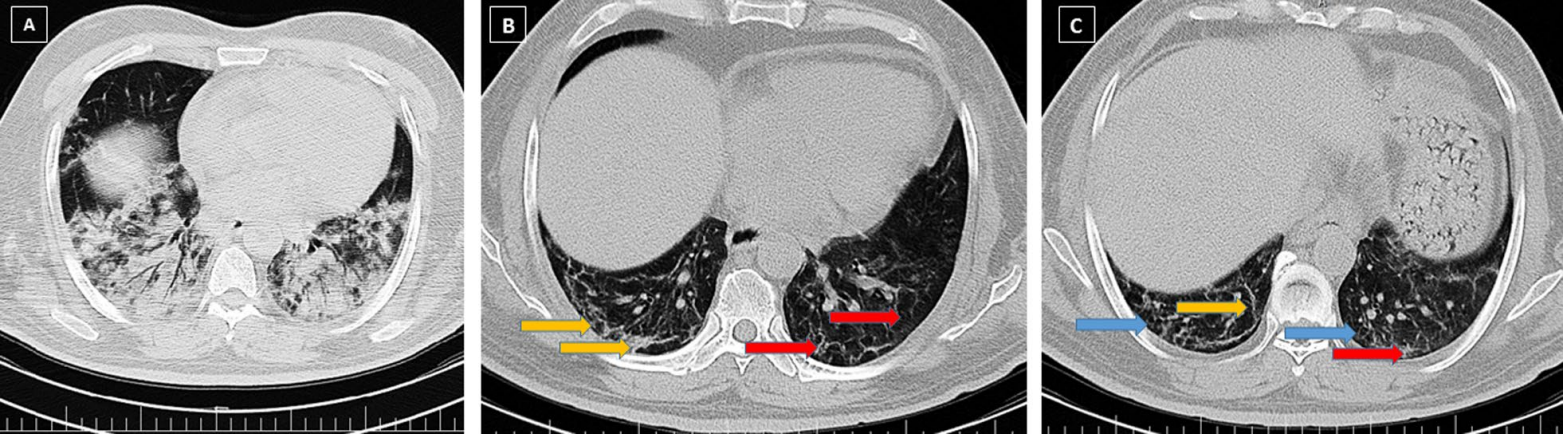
A 55-year-old male patient presented with fever, dyspnea and sputum discharge, who was admitted to hospital and was discharged on day 19 of admission. Ground glass opacities and consolidations in multiple pulmonary lobes are observed in the initial CT scan (A). The total CT severity score was calculated as 23, and the disease severity was severe. Three-month (B) and 6-month (C) follow-up CT shows parenchymal bands (yellow arrows), interlobular septal thickening (red arrows), and vacuolation (blue arrows), which were persistent findings without any significant changes after 3 months
